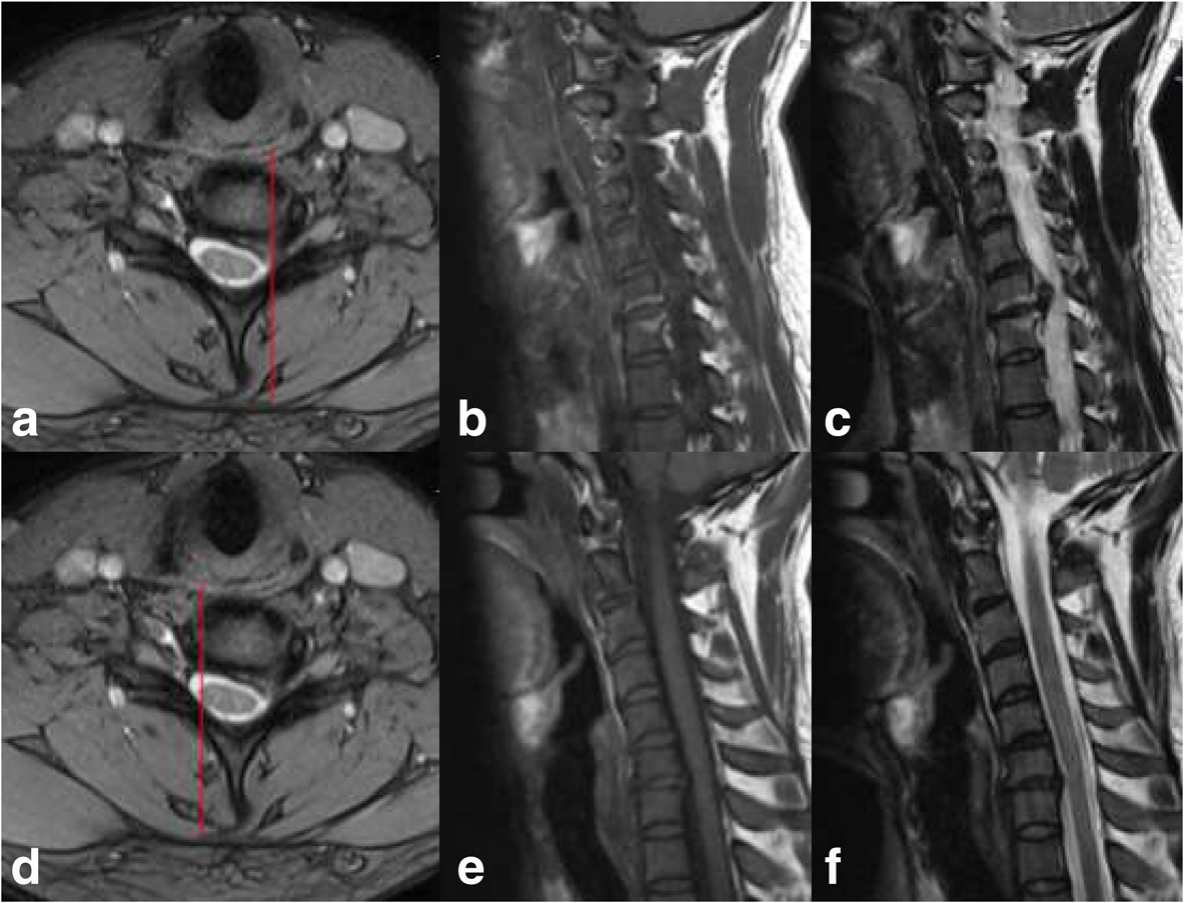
Asymmetrical Modic changes. a The axial view of MRI and the red line was the location of b and c. b, c Both T1 and T2 hyperintense on the left side of C6-7. The red line in d was the location of e and f. e, b No signal changes were found on the right side of C6-7, which indicated MCs in C6-7 were asymmetrical and unilateral
